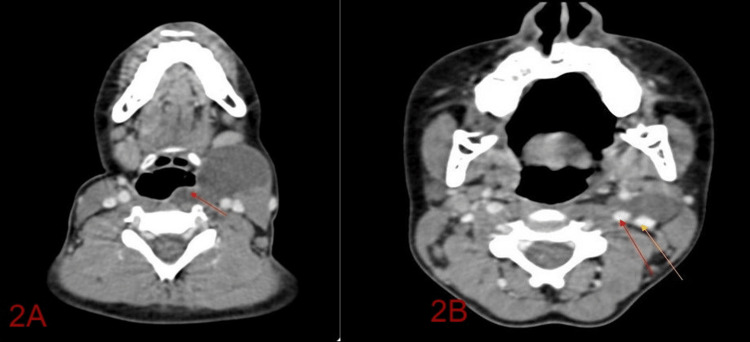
(A) Contrast-enhanced computed tomography showing cyst causing compression of the pyriform fossa (red arrow). (B) Contrast-enhanced computed tomography showing the cyst causing splaying of internal carotid arrow (red arrow) and external carotid arteries (yellow arrow).
